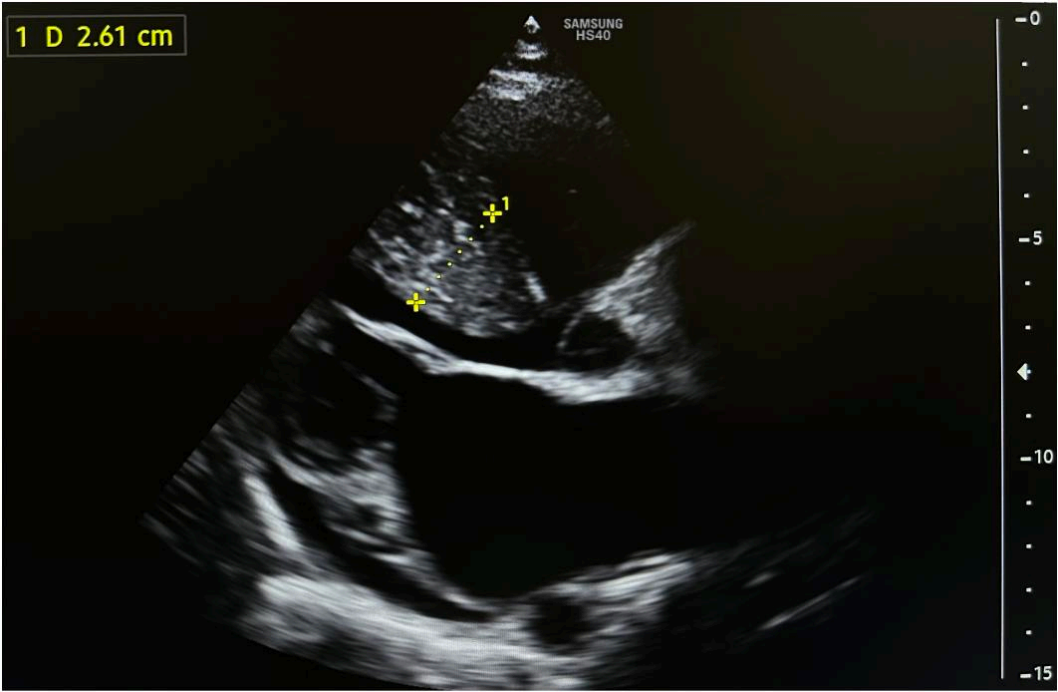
Subsequent to the first ethanol ablation, a follow-up echocardiography demonstrated a reduction in the thickness of the left ventricular (LV) septum to 26 mm, alongside a decrease in the left ventricular outflow tract (LVOT) gradient to 16 mmHg.
